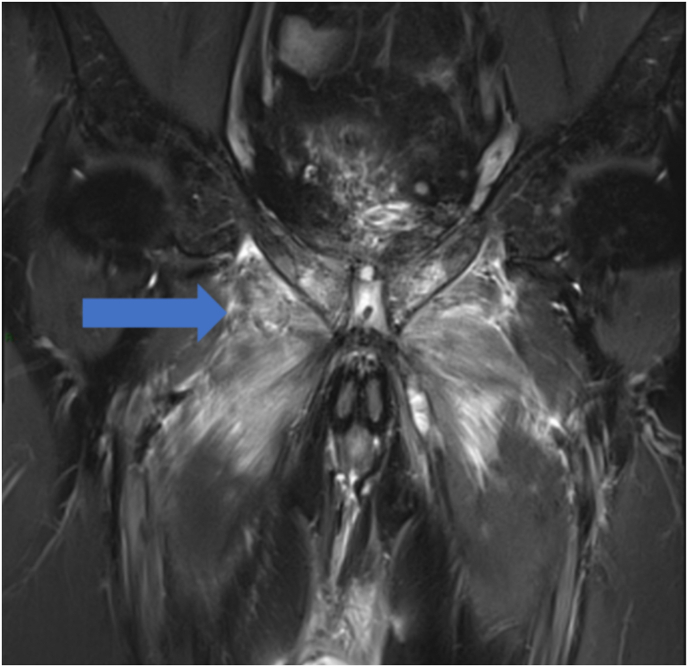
MRI, a, T2 FS-weighted coronal MRI sequence showing symmetrical edema involving the pectineus, obturator and adductor muscles.
